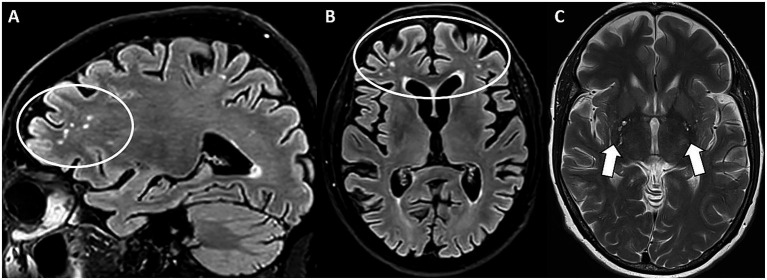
Example of MRI migraine markers. Sagittal (A) and axial (B) FLAIR and axial T2w (C) MR images of a patient with known migraine. There are confluent white matter hyperintensities [Fazekas grade 2, white circles in (A) and (B)] predominantly in frontal subcortical and deep white matter regions. Symmetrically enlarged perivascular spaces are seen in the posterior limb of the internal capsule [arrows in (C)].
